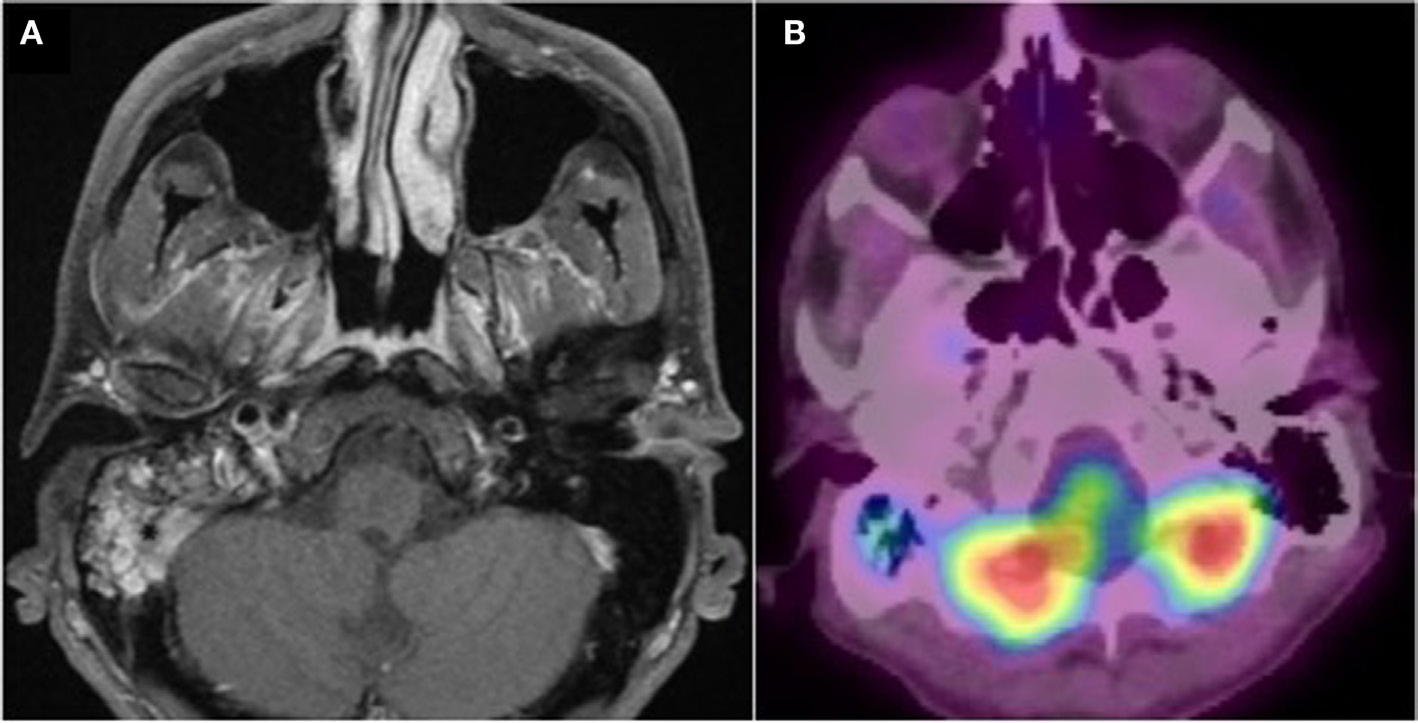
Post treatment imaging. (A) Gadolinium-enhanced axial T1 weighted fat-suppressed MRI. Minimal persistence of gadolinium enhancement in the right mastoid. (B) PET CT fused image. Good aeration and minimal persistence of 18 FDG uptake in the right mastoid. No other associated bone or tissue anomaly.
